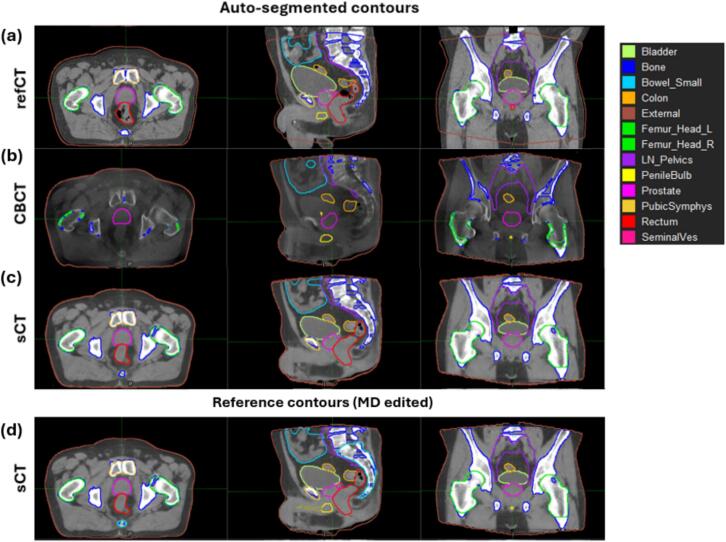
Example axial, sagittal, and coronal views of (a) refCT (Window/Level (W/L): 500/39 HU), (b) clinical CBCT (CBCT) (Window/Level (W/L): 865/16 HU), and (c) sCT (W/L: 500/39 HU) from a representative patient. The auto-segmented contours listed in the legend were generated and overlaid on the corresponding images. (d) sCT with physician-edited contours.
